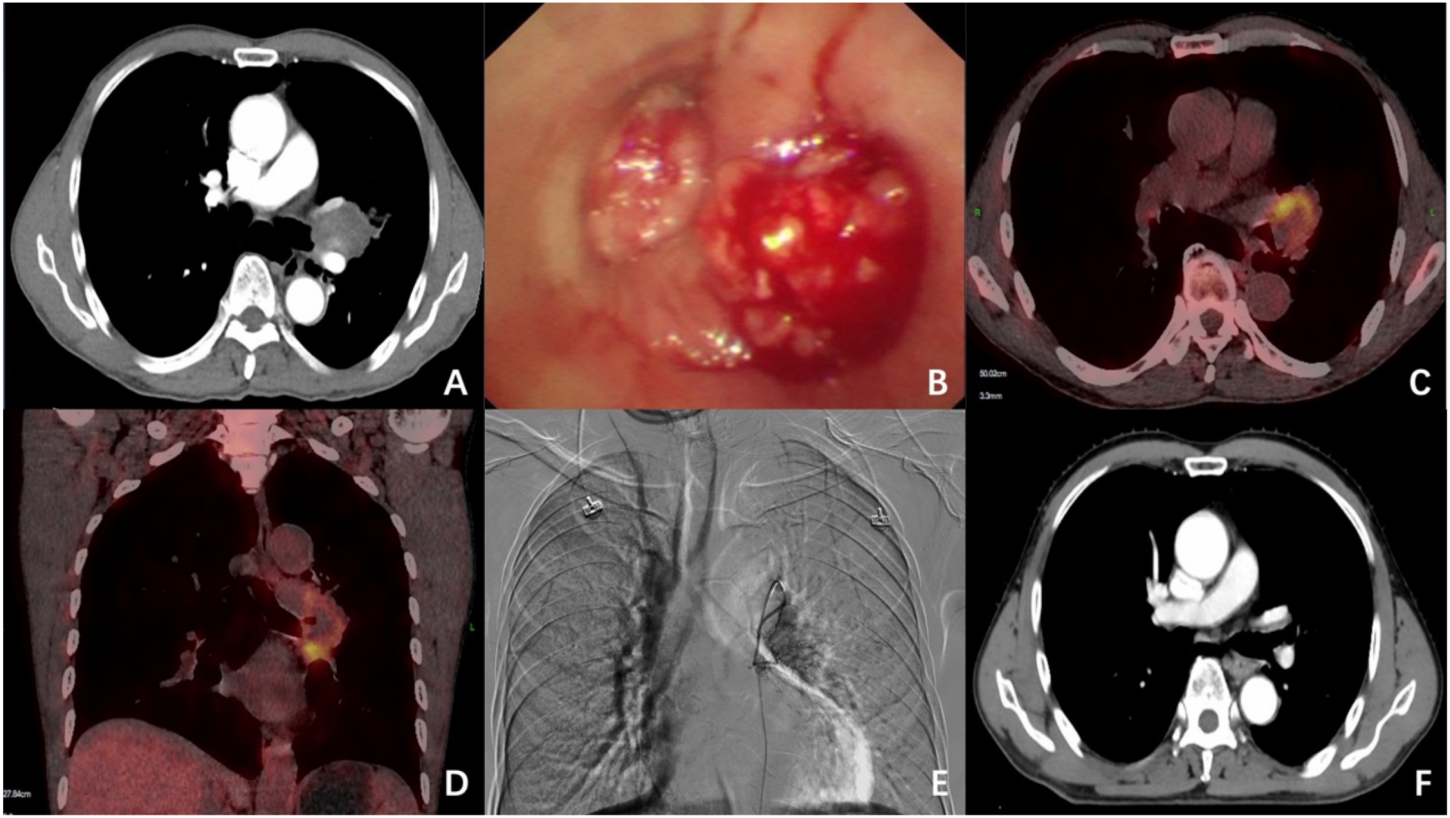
Imaging, endoscopic and DSA findings of Case 1: (A) Chest CT demonstrates a 4.1×5.2 cm mass at the left hilum. (B) Bronchoscopy reveals a neoplasm with hemorrhage obstructing the orifices of both left upper and lower lobe bronchi. (C, D) PET-CT shows markedly increased metabolic activity in the left hilar mass (SUVmax 8.3) and enlarged, hypermetabolic 10L lymph nodes (SUVmax 8.1). (E) DSA reveals hypertrophied bronchial arteries supplying the tumor, which exhibits rich vascularization. (F) Follow-up chest CT at 1-month post-combination therapy demonstrates complete tumor resolution.
